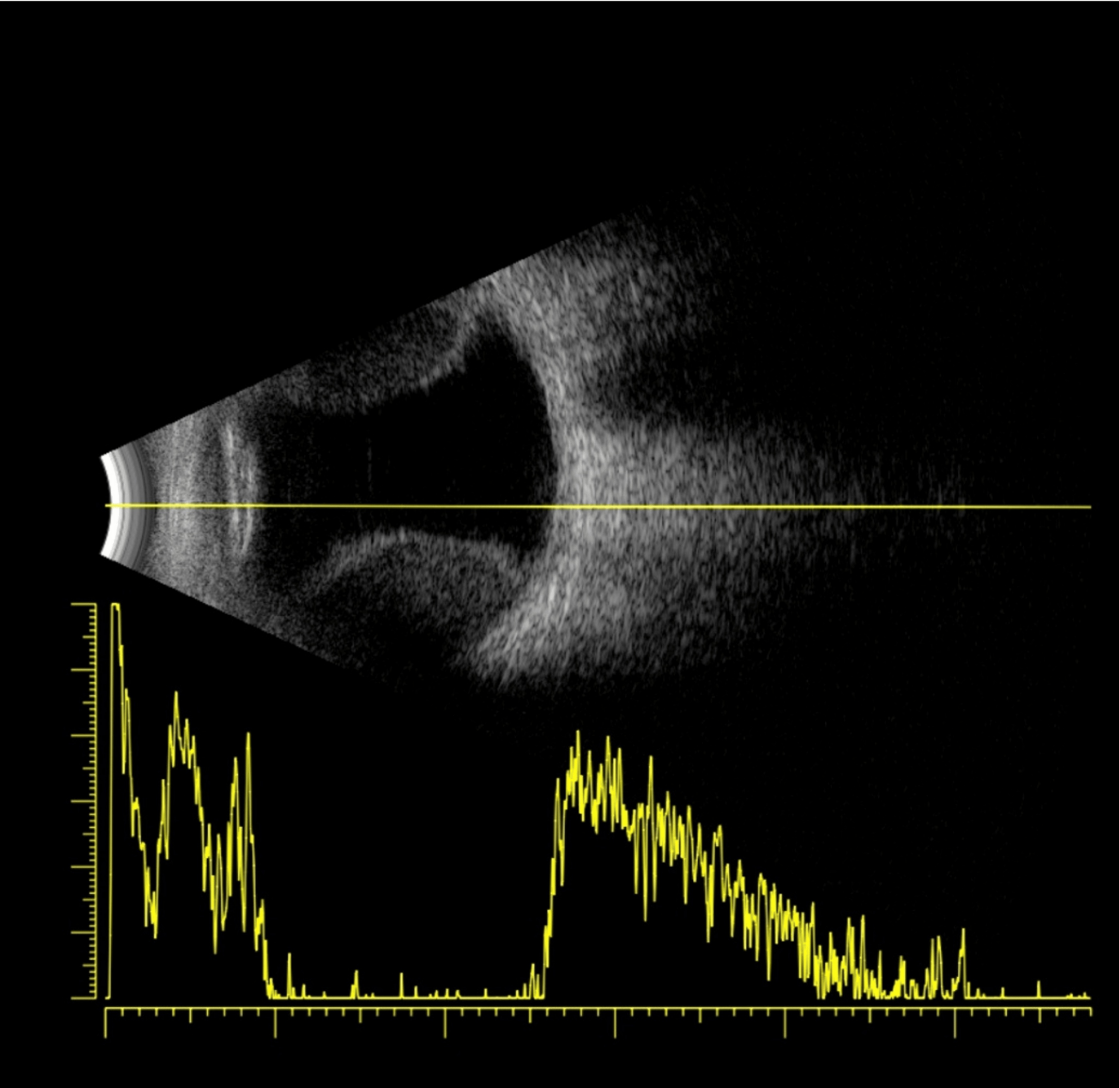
Ultrasound image showing choroidal detachment
